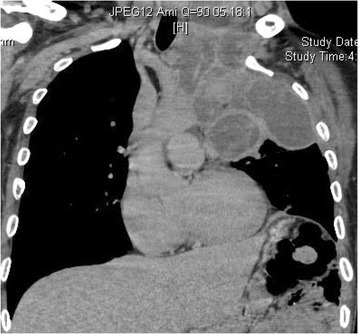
Coronal contrast-enhanced computed tomography of the neck and chest demonstrating a large multiloculated mass
